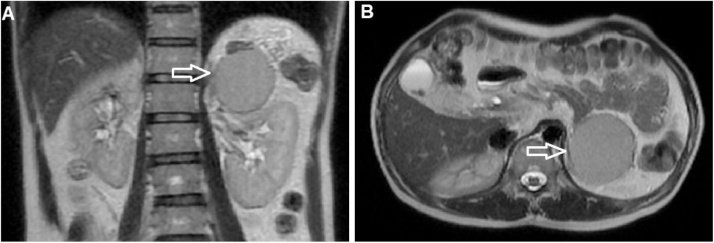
Coronal T2-weighted image (A) and axial T2-weighted image (B). Coronal T2-weighted image showed that the mass was located in the normal spleen site (arrow) and compressed the left kidney. The mass was well-circumscribed and had a higher signal than liver on axial T2-weighted image (B, arrow).
